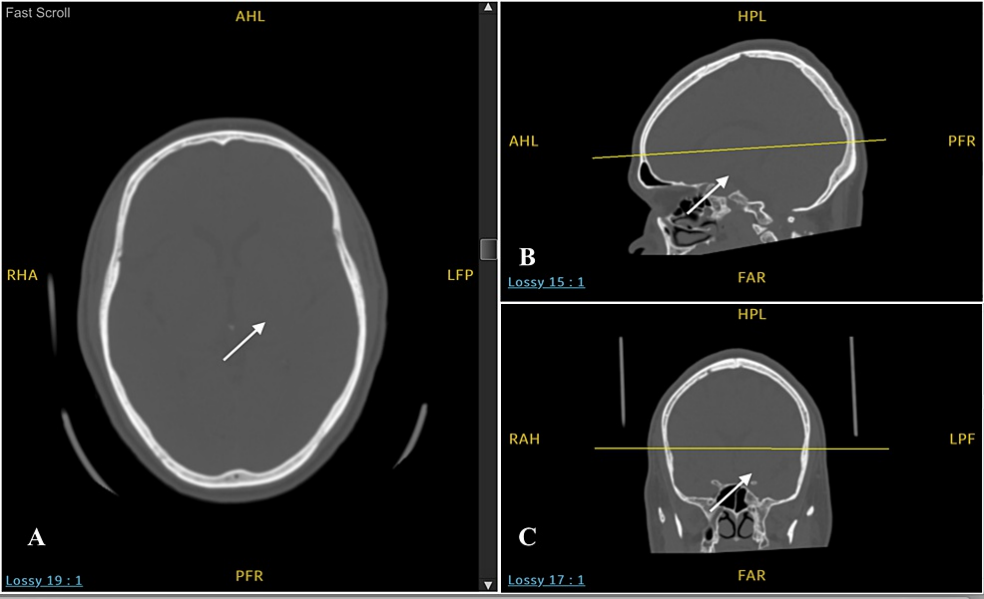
CT brain without contrast in the (A) axial (B) sagittal and (C) coronal planes showing (arrow) old infarcts in the left basal ganglia.
